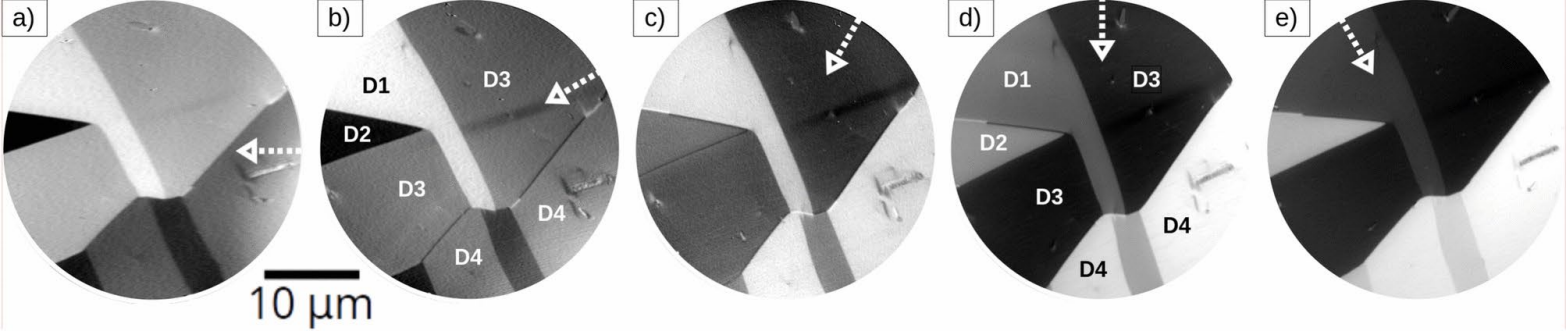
XMCD-PEEM images of the same area of the magnetite (110) surface, acquired at the Fe L$$_{3}$$ edge, at different azimuthal angles, of 0$$^\circ$$, 30$$^\circ$$, 60$$^\circ$$, 90$$^\circ$$ and 120$$^\circ$$. The images have been rotated back by the same amount in order to identify the same features. The incoming x-ray beam direction is marked by an arrow in each image.
